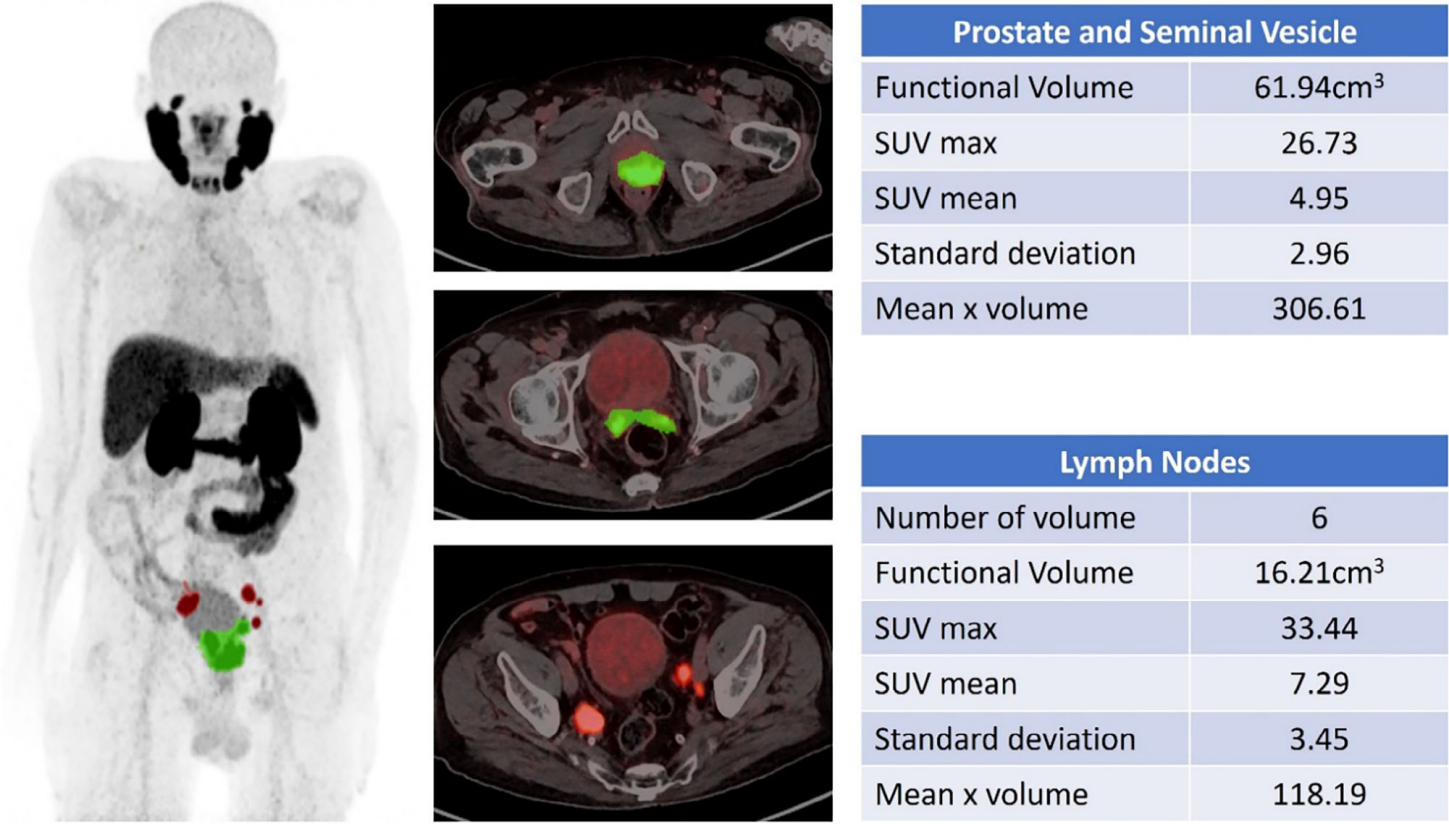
^68^Ga‐PSMA PET‐CT showing prostate lesion extending to seminal vesicle. Multiple ^68^Ga‐PSMA avid pelvic lymph nodes are observed. No evidence of distant metastasis.
